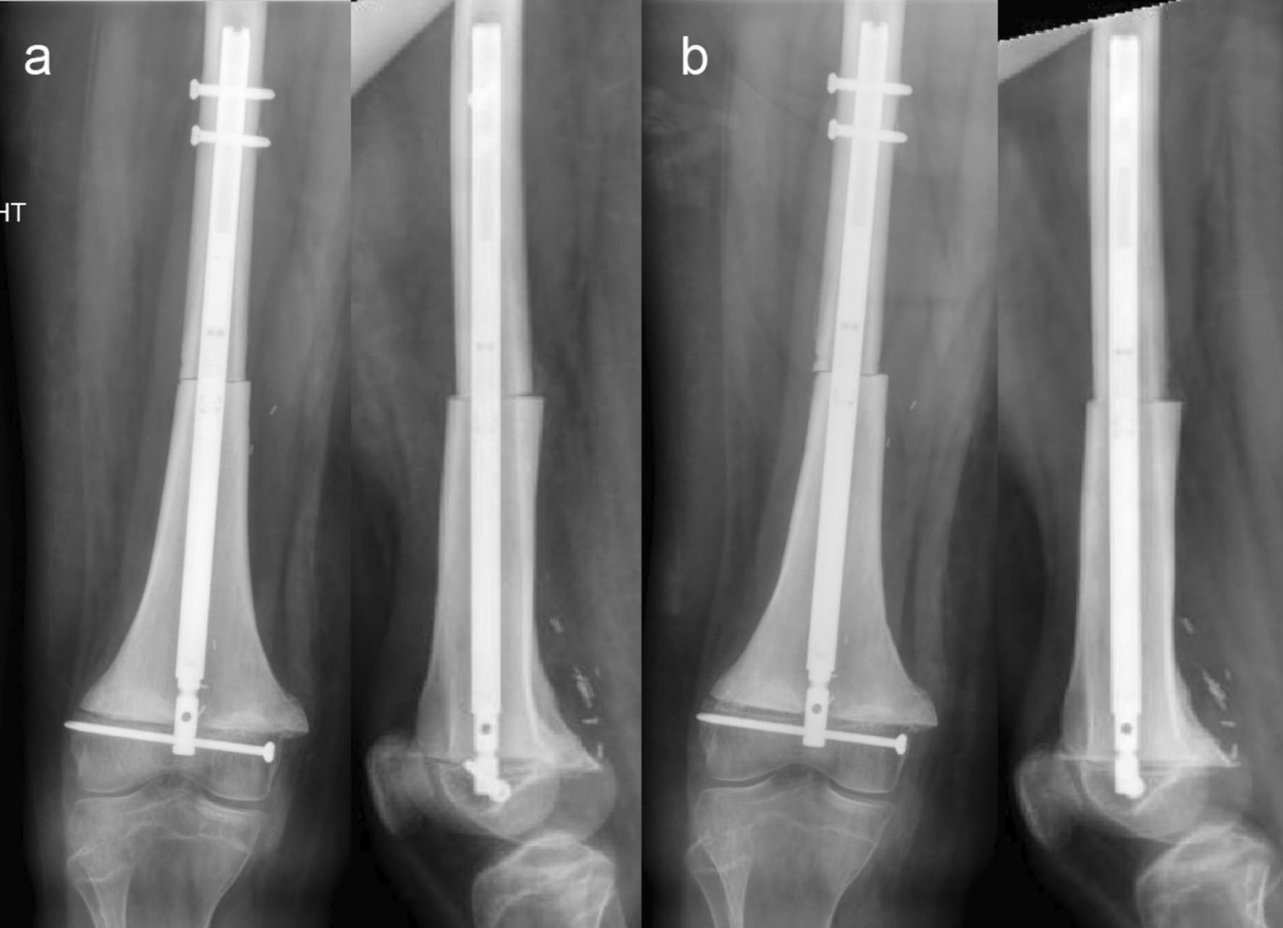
Anteroposterior and lateral radiographs of the distal femur after tumor resection and allograft reconstruction undergoing compression prior to MRI (a). Anteroposterior and lateral radiographs immediately after the MRI demonstrate no evidence of activation of the nail or hardware failure (b)
